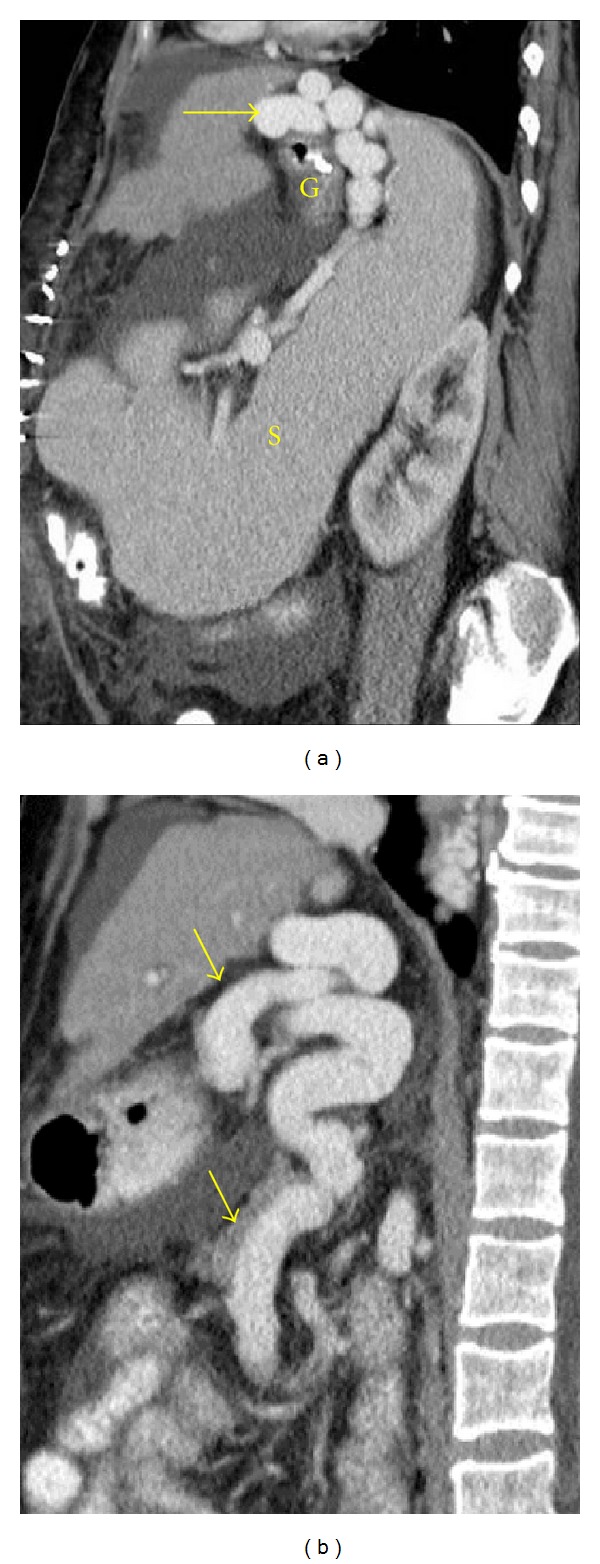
Splenomegaly in a cirrhotic patient with portal hypertension. Sagittal contrast-enhanced CT images ((a)-(b)) show an enlarged spleen (S) associated with retrogastric varices (long arrow) and splenorenal shunt (short arrows). G: gastric fundus.
